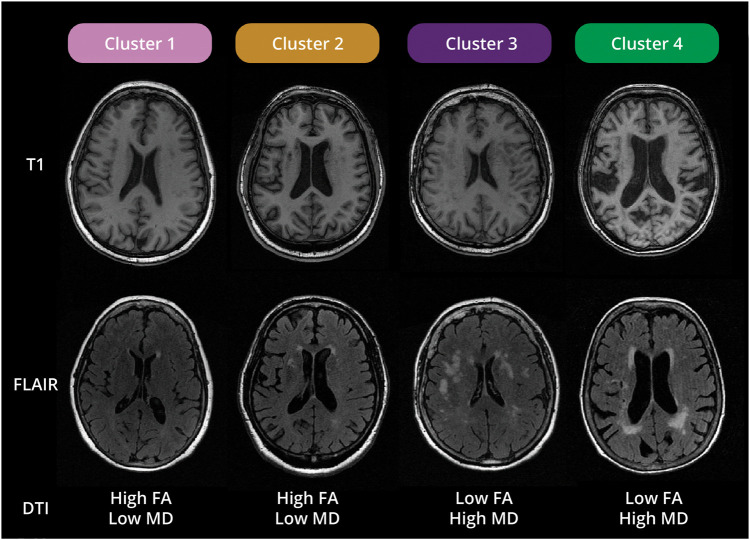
Typical MR Images of the Brain for Each Cluster For each cluster, MR images of a single participant in that cluster are shown to demonstrate typical MR images of the brain for each cluster. Microstructural properties derived from DTI were shown as text rather than images since diffusion-weighted images for fractional anisotropy and median diffusivity are difficult to interpret intuitively. DTI = diffusion tensor imaging; FA = fractional anisotropy; FLAIR = fluid-attenuated inversion recovery; MD = mean diffusivity.
